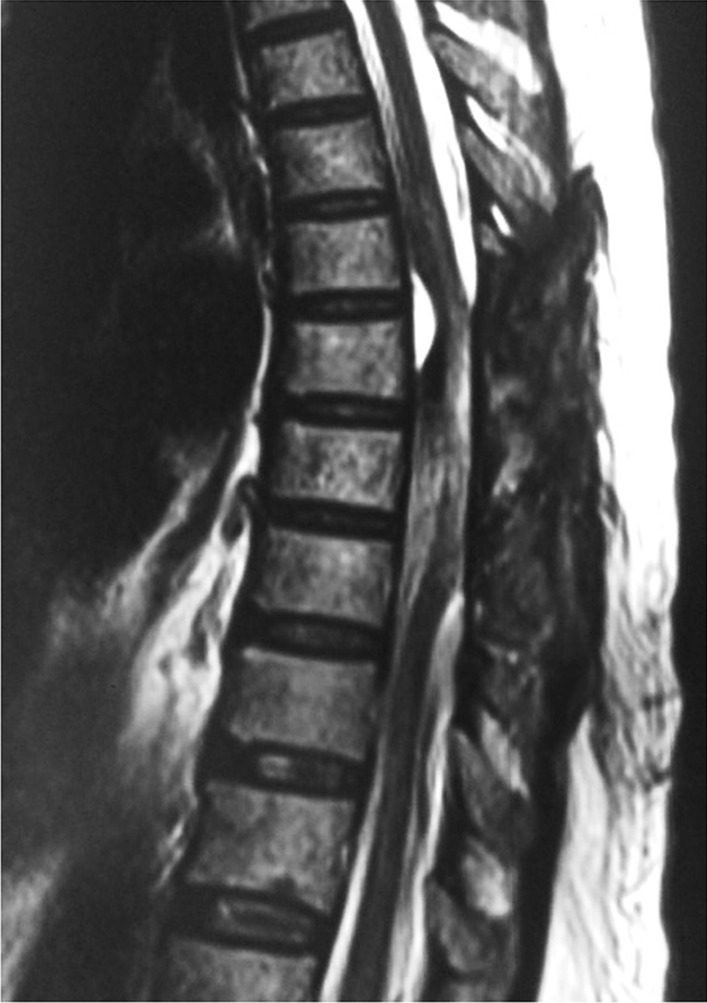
Postoperative T2-weighted sagittal image showing small hematoma in the anterior epidural space with cord edema at D7–D8 vertebral level
